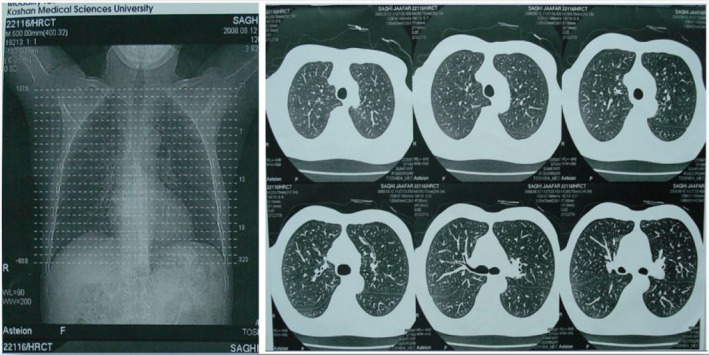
The thorax HRCT scan: No evidence of mass lesions and airway diseases were observed in the HRCT scan
